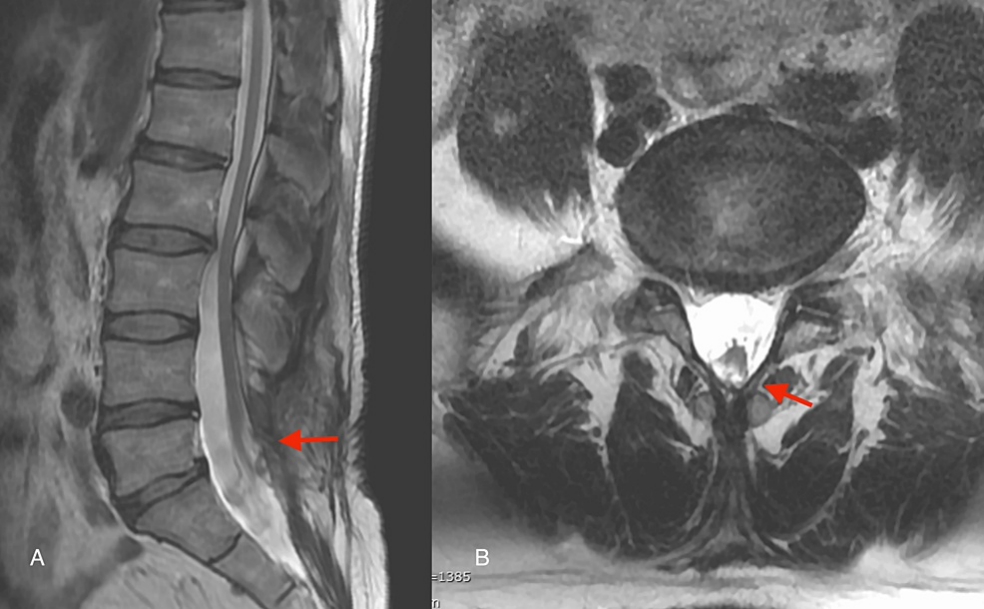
Preoperative imaging (A) The sagittal MRI showed that the descending spinal cord cone was contiguous with a subcutaneous lipoma (arrow). (B) The axial MRI shows a dorsal displacement of the spinal cord (arrow).
